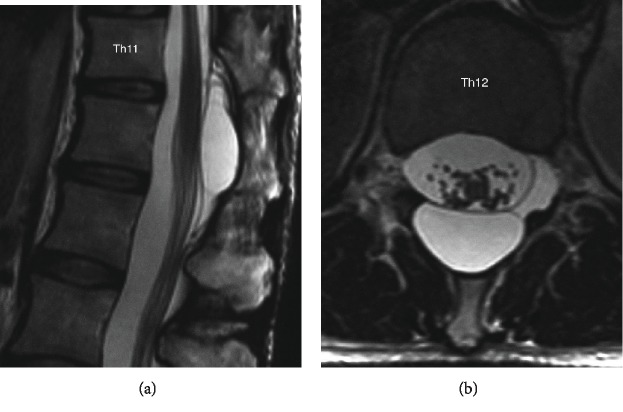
T2-weighted MRI showed a cystic lesion with T1-low/T2-high intensity at the T11-L1 level.
